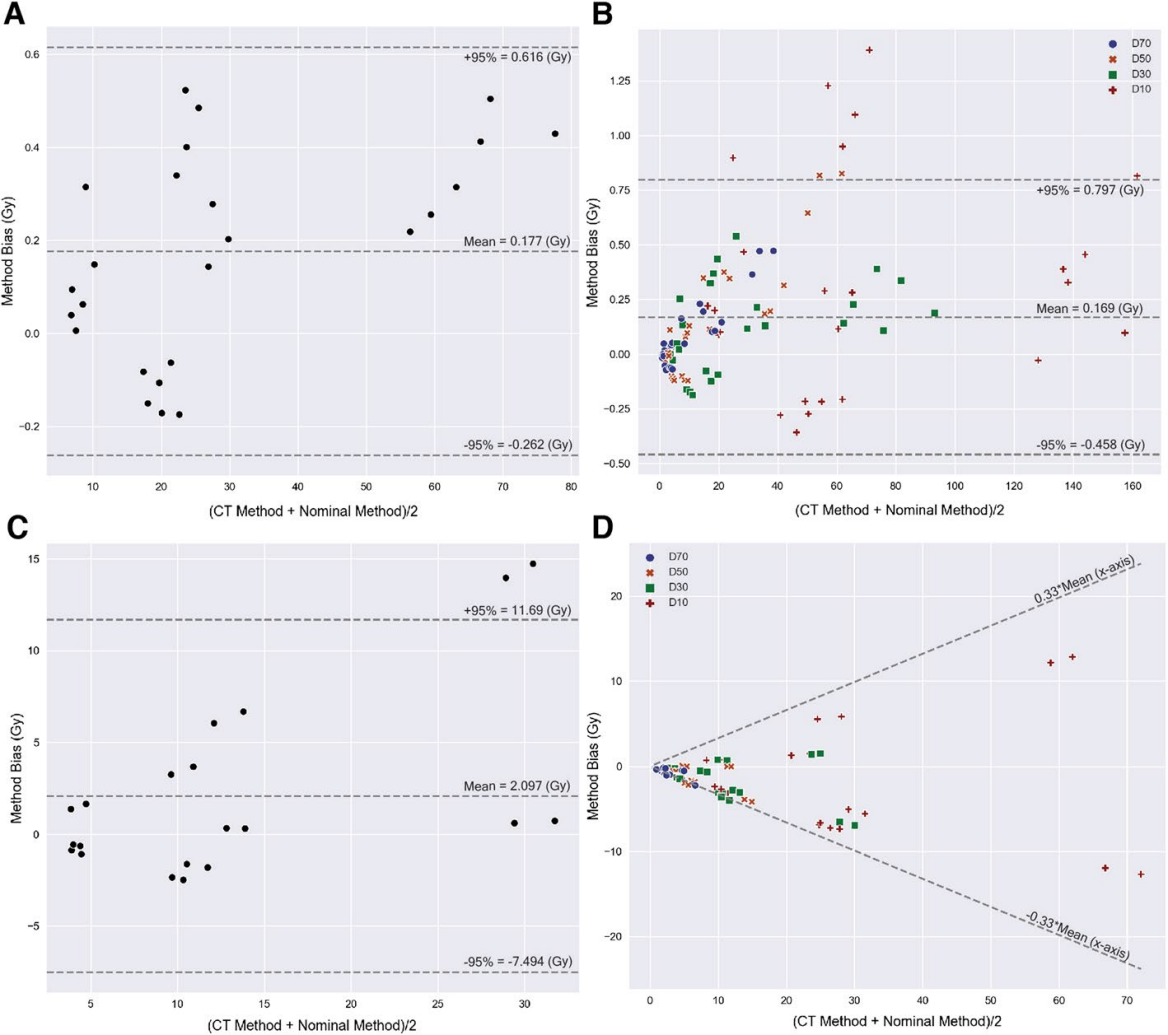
The Bland Altman analysis plots the mean bias and 95% CIs between the CT and nominal local deposition methods for the mean doses (A, C) and all the cumulative dose volume histogram (DVH) dose metrics (B, D). Parts A, B refer to the liver while C, D refer to the lungs. Parts A, C compare between every self-calibration mean dose estimate for the liver $$N=25$$ and the lungs, $$N=20$$. Parts B, D compare between every self-calibration DVH dose metric in the liver $$N=100$$ and the lungs $$N=80$$. *N* represents the number of data points compared for each Bland Altman plot. The lungs did not include the Liver calibration
